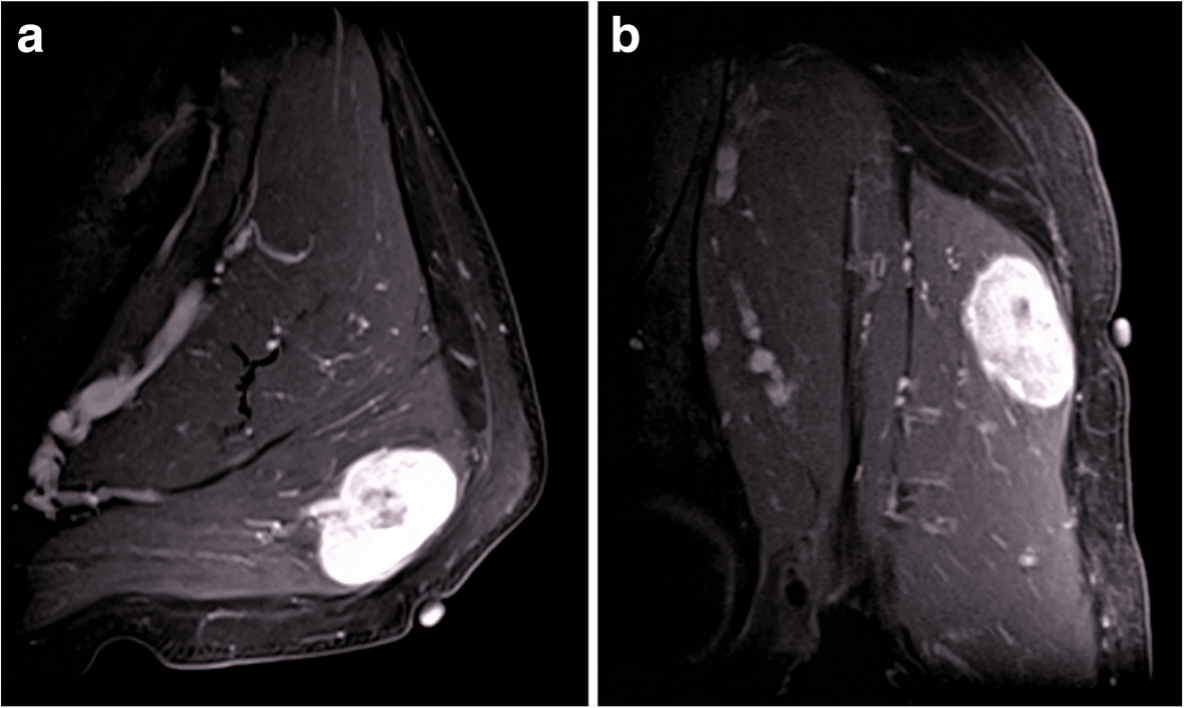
Magnetic resonance findings. a The axial T1-weighted image with intravenous gadolinium enhancement showed irregular peritumoral enhancement. b The sagittal enhanced T1-weighted image demonstrated a poorly enhanced region at the center of the tumor
